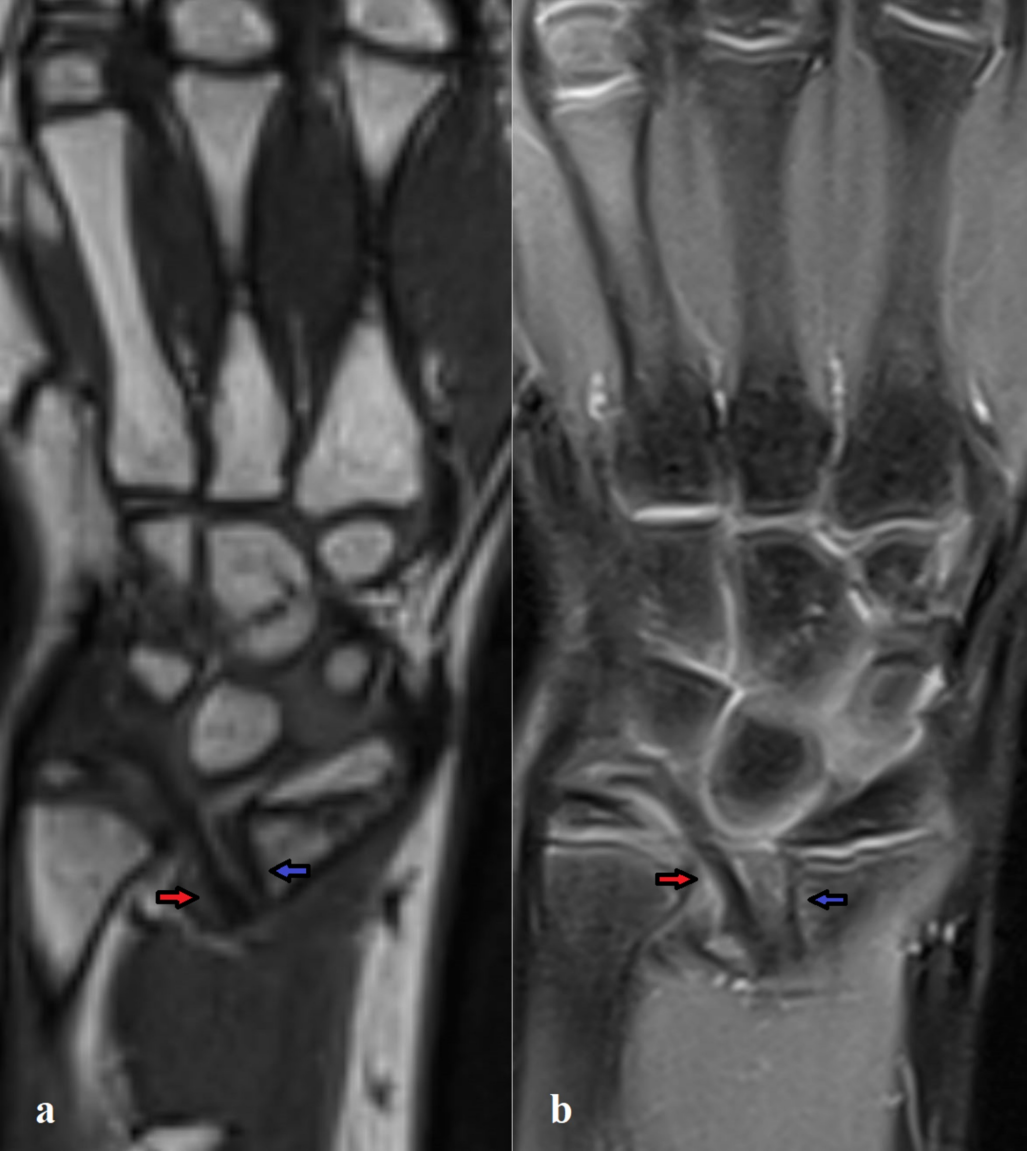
MRI aspect of the VL and RTL. Visualization of VL (blue arrow) and RTL (red arrow) on T1-space isotropic coronal MRI (a) and PD-tse fat suppressed coronal MRI (b). VL appears as an abnormal volar radiolunate ligament short and thickened. RTL is another abnormal volar ligament long and thickened just lateral to the VL. VL, Vickers ligament; RTL, radiotriquetral ligament; PD-tse, proton density-turbo spin echo
